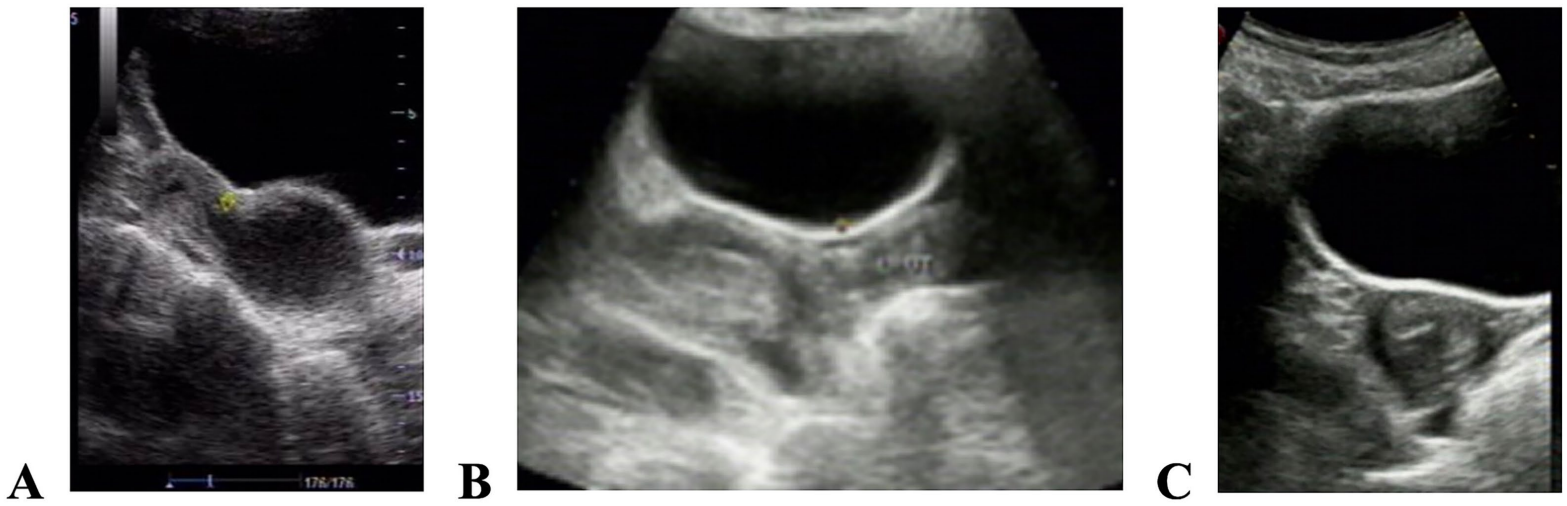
Pelvic ultrasound images before and after surgical correction of oblique vaginal septum syndrome, complicated by a rotated uterus. (A) Preoperatively, the right uterine myometrium showed a homogeneous echotexture, and separation of the uterine cavity was approximately 1.8 cm; an anechoic area measuring ~7.5 cm × 5.0 cm was detected inferior to the uterus, containing punctate weak echoes and communicating with the uterine cavity; (B) Postoperatively, the right uterine body measured ~4.5 cm × 2.2 cm and the left uterus measured ~3.9 cm × 2.0 cm; continuity between the endometrial line and the cervical canal was good bilaterally; on downward scanning along both uterine bodies, enlargement was observed at the confluence; (C) On transverse ultrasound scanning from the uterine body to the cervix and vagina, the two cervices showed a fused configuration inferiorly; the right cervix gradually shifted to the right anterior position, and the left cervix was located in the left posterior position.
